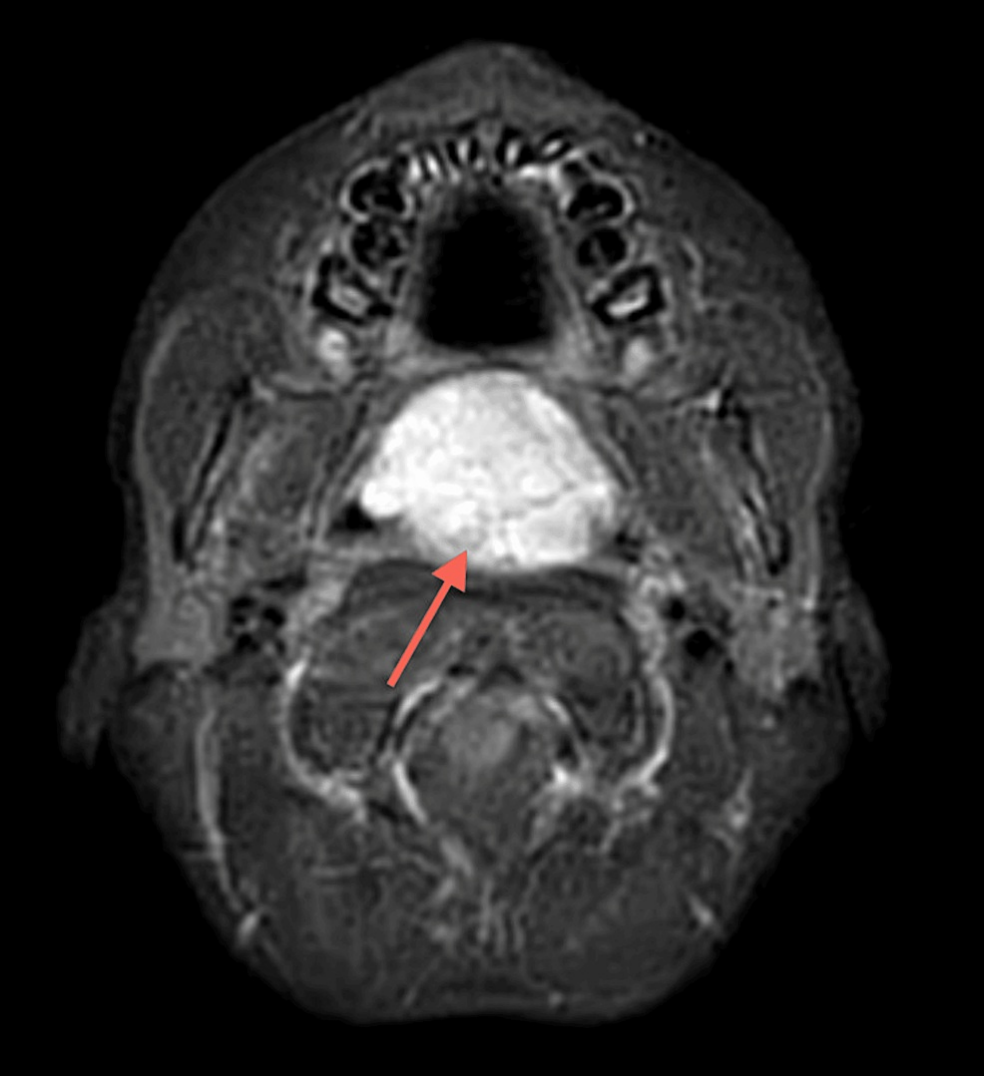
MRI Neck (Post Contrast) The large nasopharyngeal well-circumscribed cyst measures 47 x 28 x 35 mm. The sheer volume of this Thornwaldt cyst is remodeling the undersurface of the sphenoid sinus. The cyst is anteriorly eroding the posterior edge of the hard palate and midline vomer segment of the nasal septum, the uvula is displaced significantly into the oral cavity. Parapharyngeal recesses are preserved. No intracranial extension.
